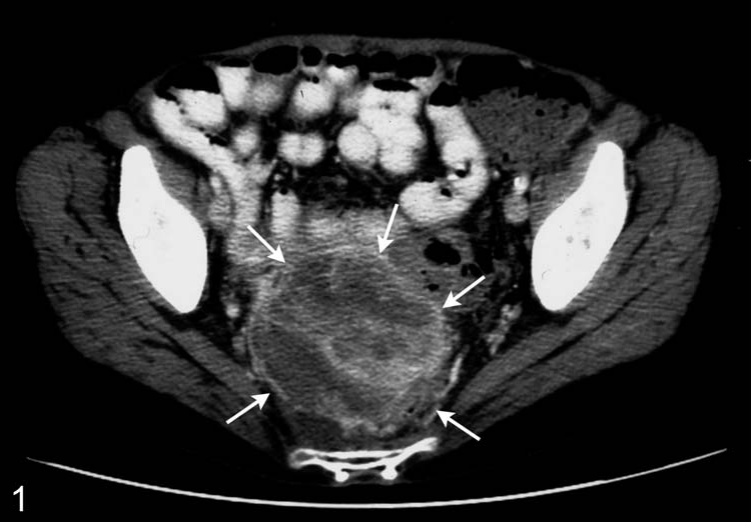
CT scan showing the presence of a large, heterogenous, solid/cystic serosal based pelvic mass (arrows).
